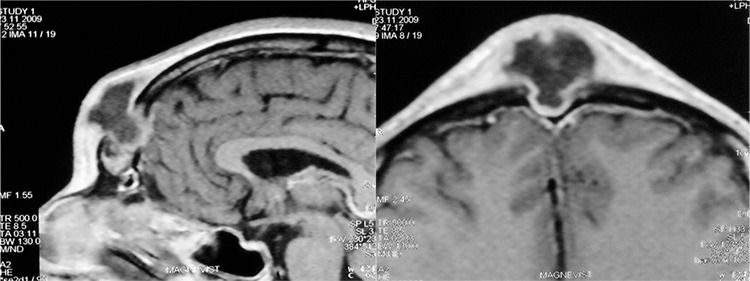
The T2-weighted sagittal (a) and axial (b) MRI images show frontal Pott’s puffy tumour with epidural-cutaneous fistula. Note the absence of other intracranial complications.
